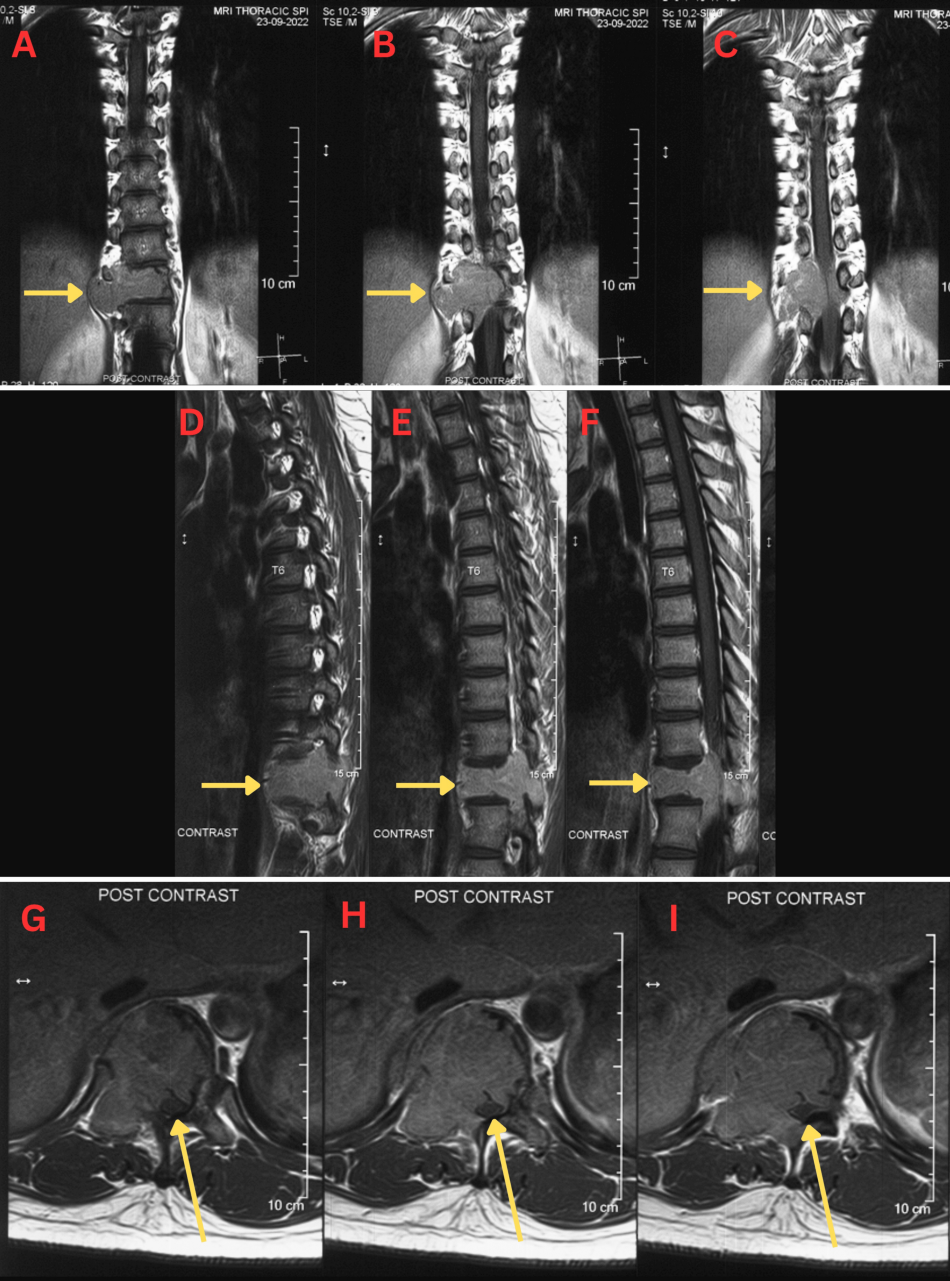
Preoperative T1 post contrast MRI of the thoracic spine in coronal (A,B,C), saggital (D,E,F), and axial (G,H,I) views. The arrows show the lesion on the T11 vertebral body extending towards the right pedicle and posterior element. The mass effect has narrowed the spinal canal and pushed onto the spinal cord, as seen in arrows in the axial (G, H, I) view.
